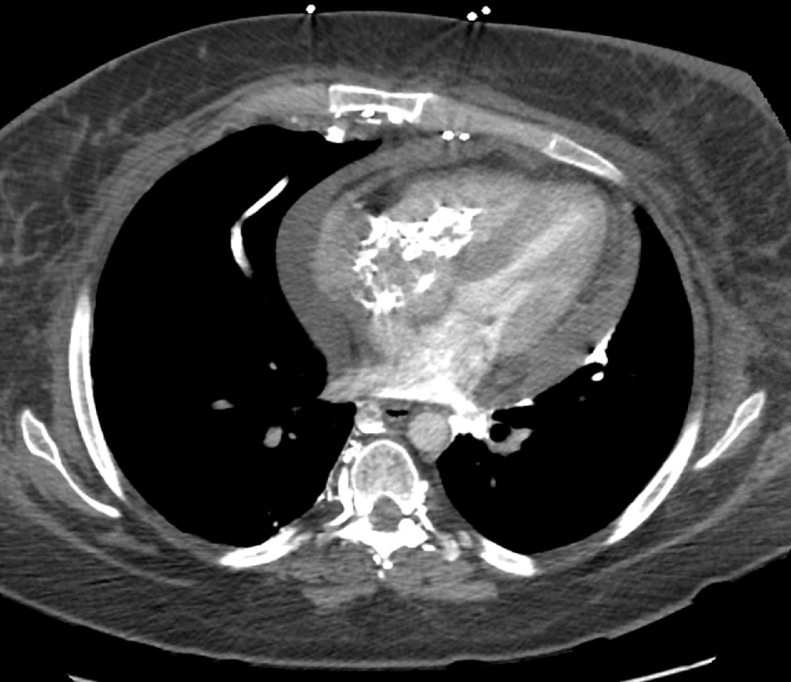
CT chest PE protocol demonstrated a large intracavitary heterogeneous irregular calcified mass extending into the right atrium, across the tricuspid valve, and into the right ventricle and pulmonary outflow tract. Additionally, small to moderate pericardial effusion was noted, with reflux of contrast in the collateral veins, suggestive of restrictive cardiac dysfunction.
